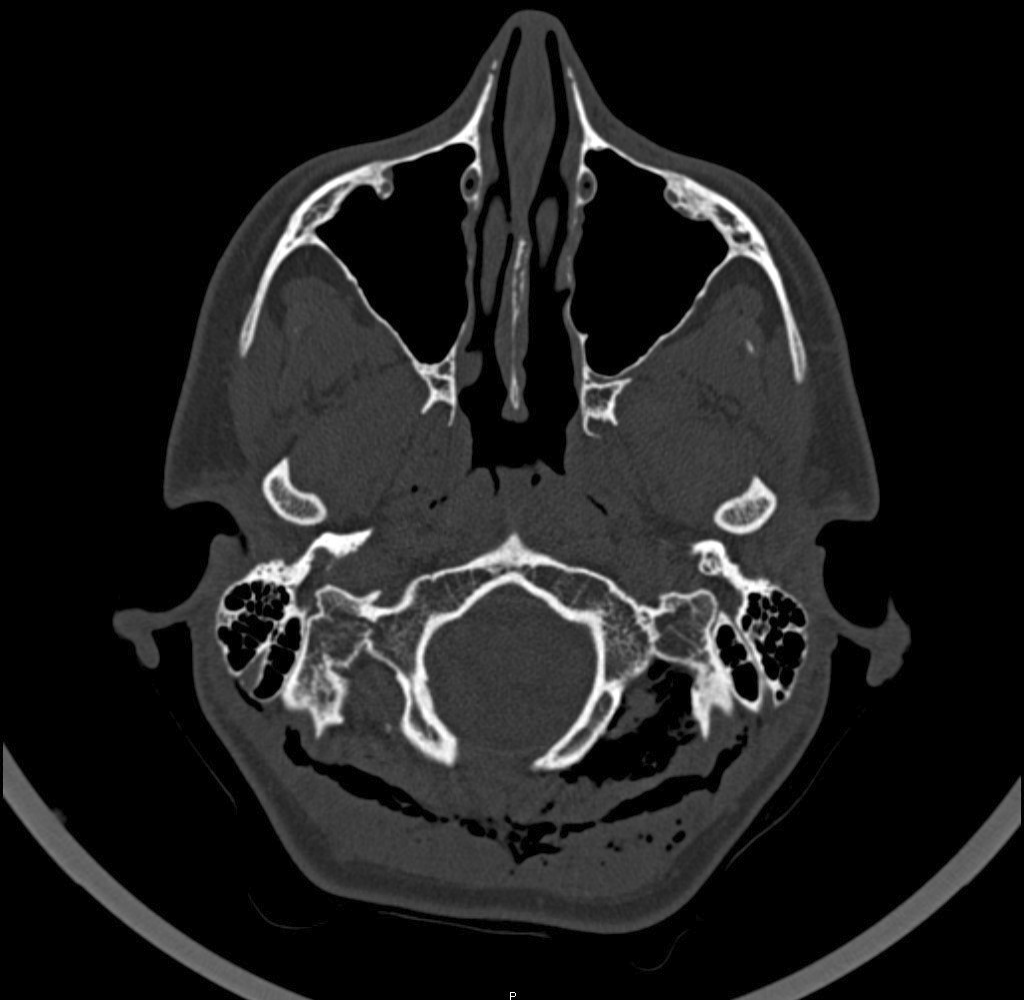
Axial plane HRCT scan – bilateral SCE evident at the level of C1, between the suboccipital muscles bilateraly
